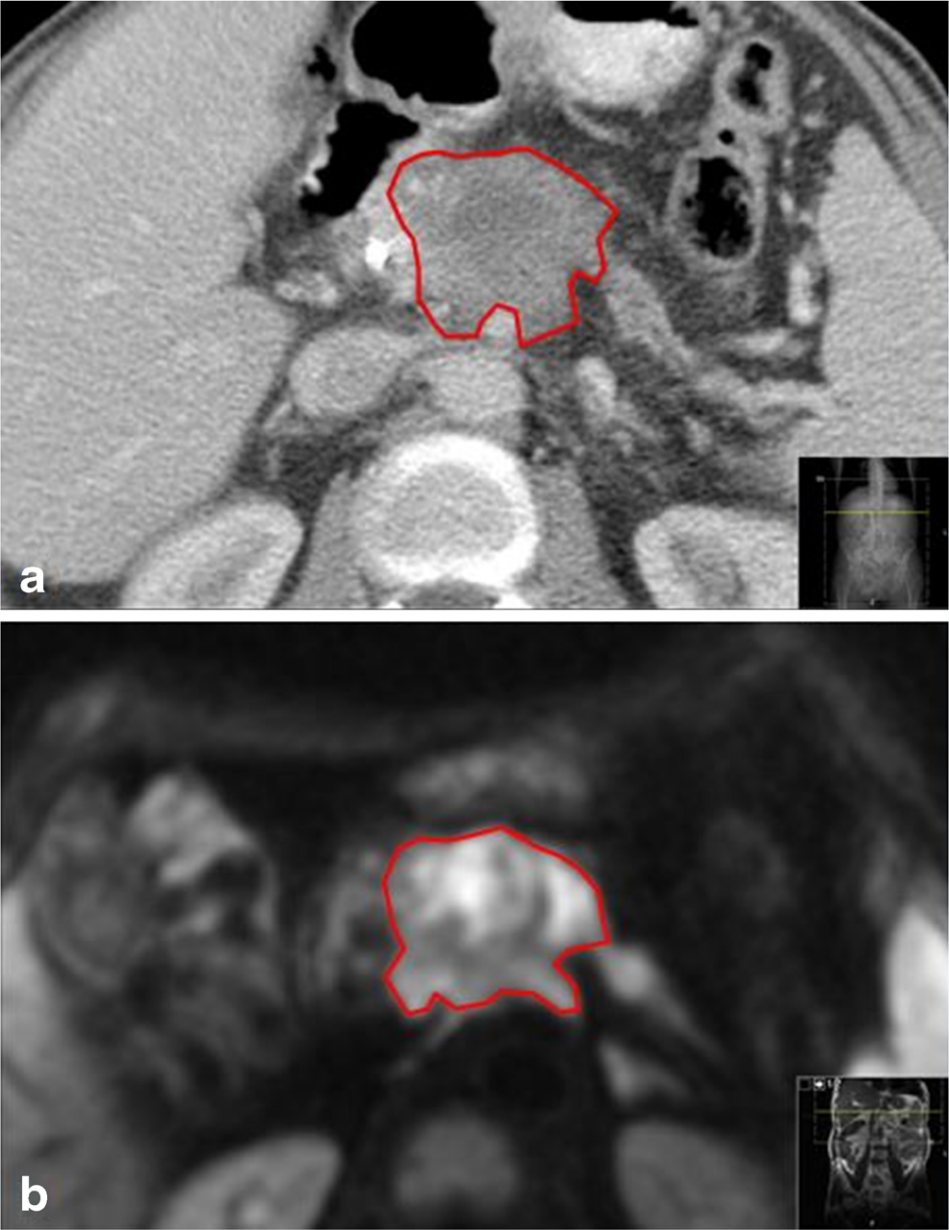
Manually drawn boundary of tumour on (a) ceCT (portal venous phase) and (b) DWI-b800 sequence
